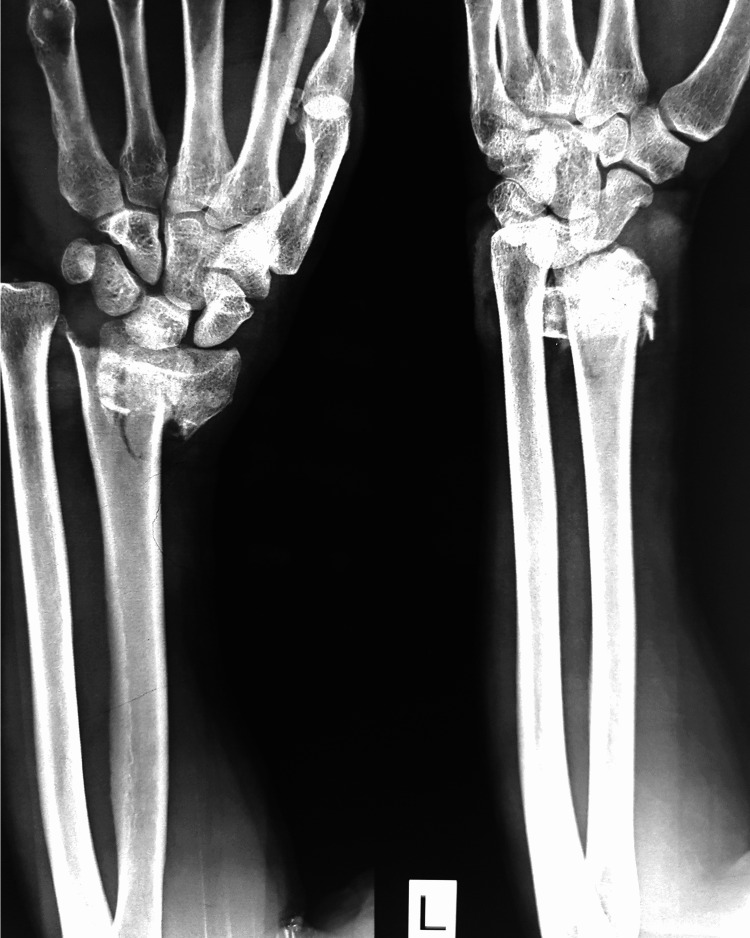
X-ray of a distal radius fracture before management
